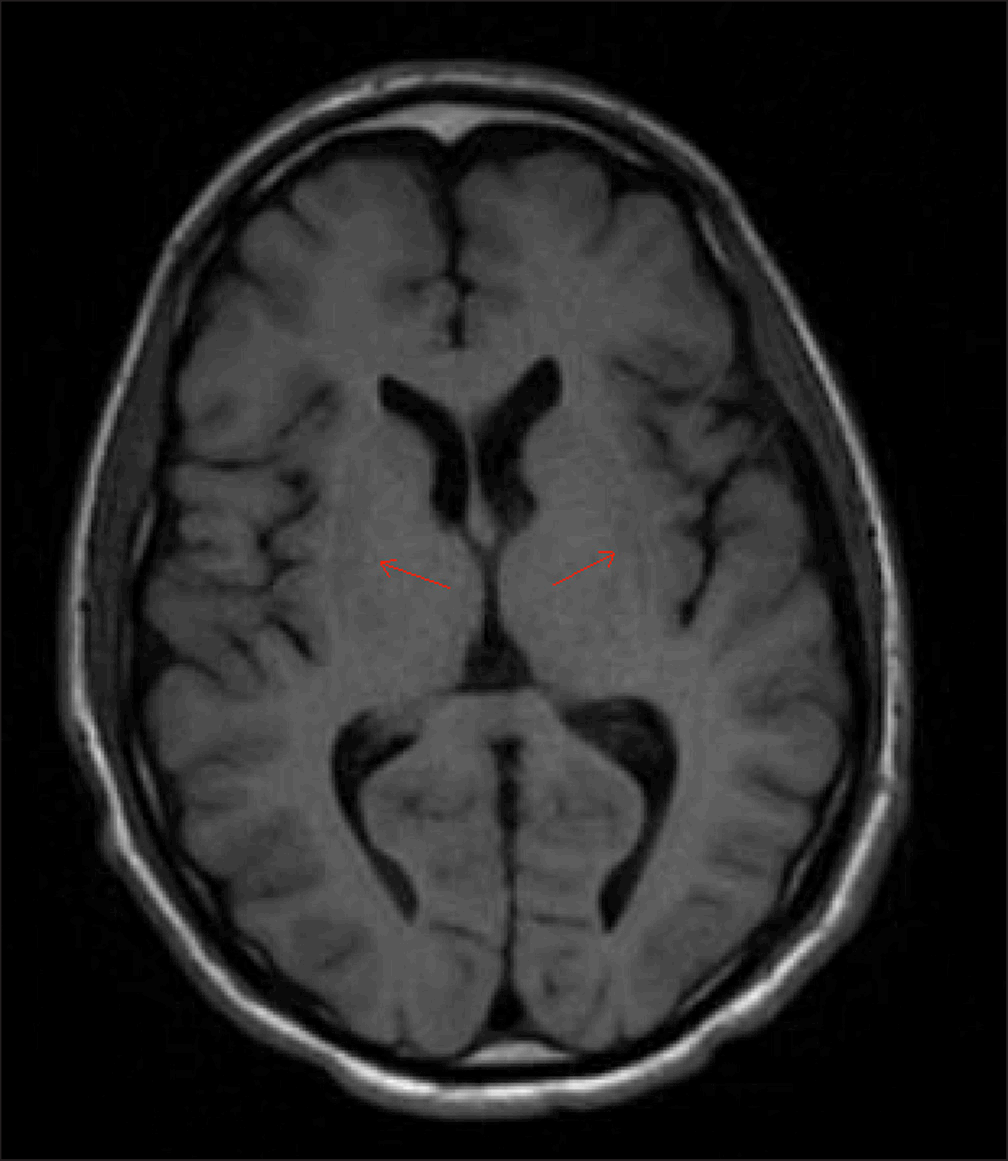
Neuroimaging in ODS. Axial T1-weighted brain MRI of the patient showing hypointensities of the bilateral lentiform nuclei (red arrows).
